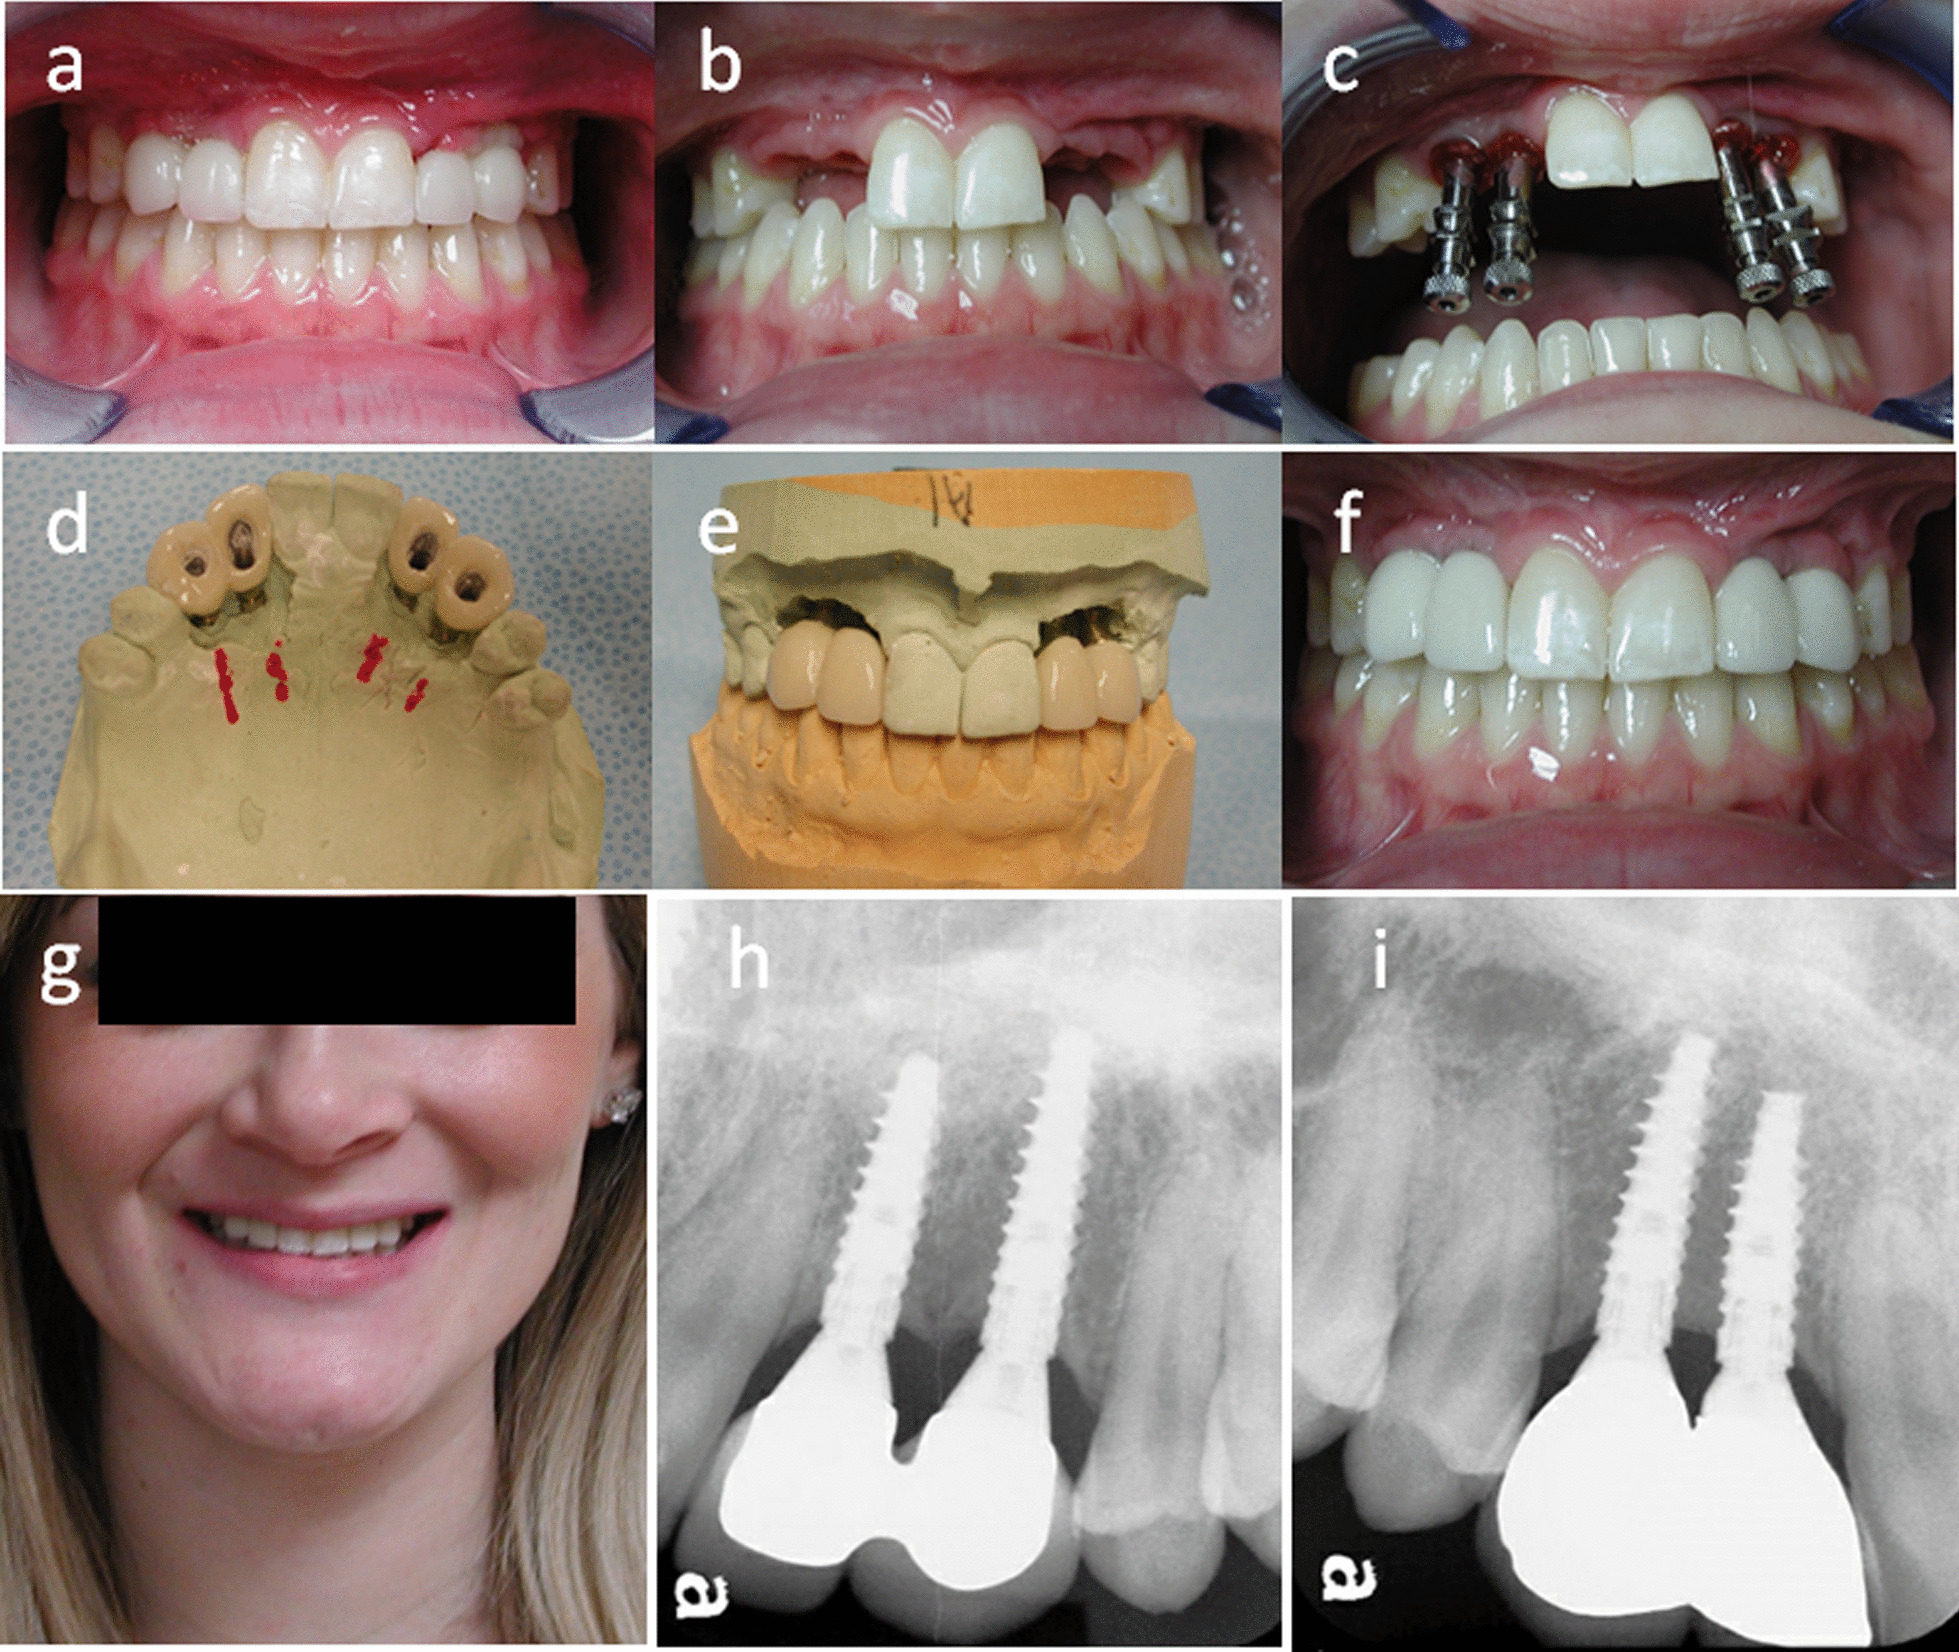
Prosthodontic rehabilitation: a Provisional prostheses: notice the gingival level was very low at initial stage. b Transmucosal tissue shaped by provisional prosthesis. c Implant level impression was taken with custom impression coping. d and e Screw-retained definitive prostheses on cast. f Intraoral view of final prostheses. g Extraoral view at the end of treatment. h and i Periapical radiographs in 3-year follow-up
